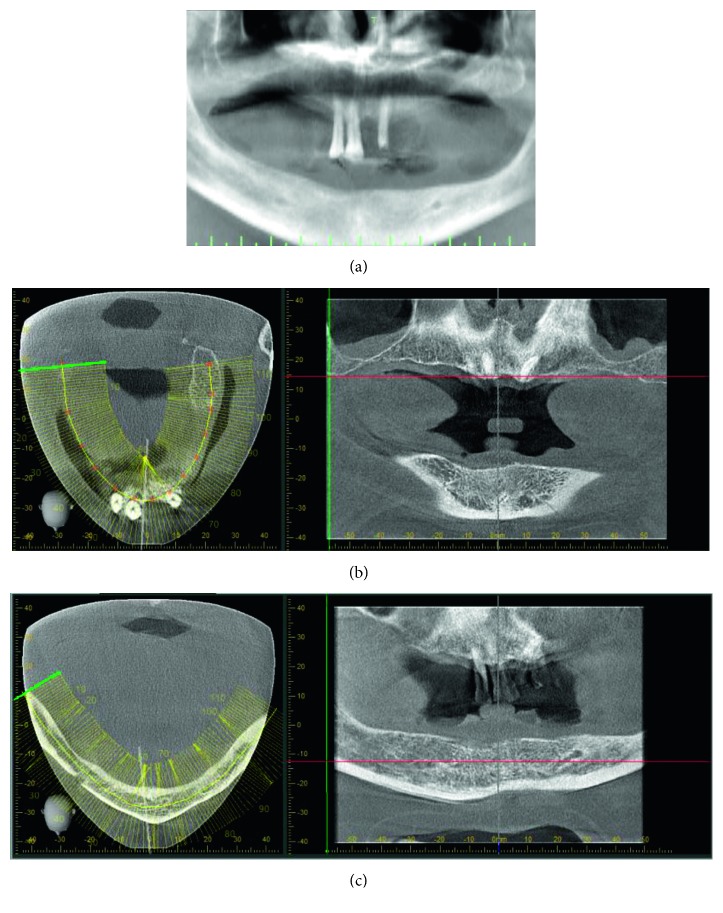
Views of preliminary CBCT evidencing sufficient bone volume in the anterior maxilla and poor bone in the lower jaw except for the interforaminal area: (a) panorex reconstruction; (b) maxilla view; (c) mandible view.
